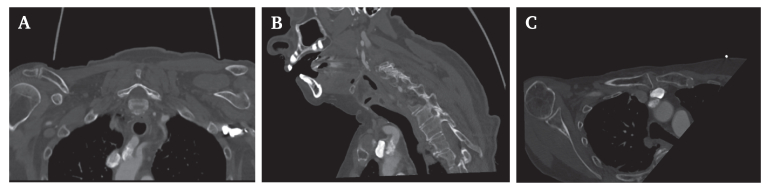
Computed tomography scan showing brachiocephalic artery lesion stenosis. (A) Coronal. (B) Sagittal. (C) Axial.
